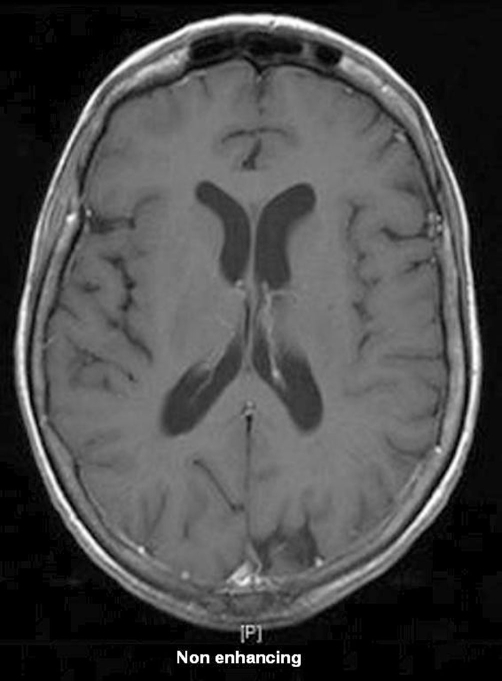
Magnetic Resonance Imaging showing nothing remarkable pre enhancement.
